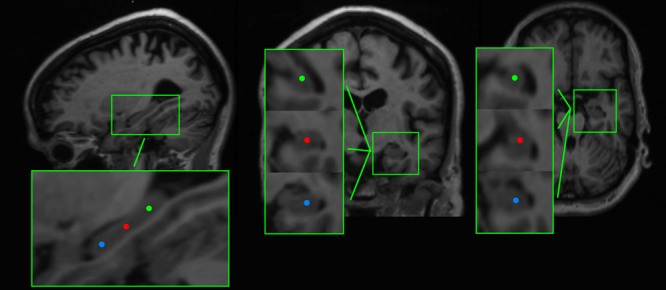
Example of the placement of virtual electrodes in one patient in the right hippocampus on T1 weighted magnetic resonance imaging (MRI). The virtual electrodes are shown as blue (anterior), red (center), and green (posterior) dots on sagittal, coronal and axial brain slices (left, middle, and right panel, respectively).
